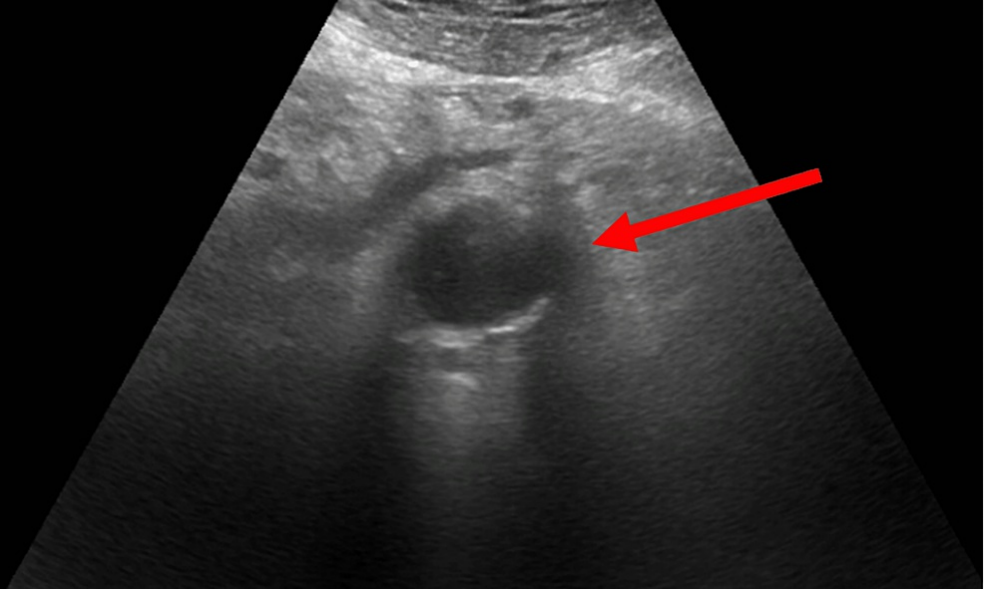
Sonogram depicting an asymmetric outpouching of the aortic wall without an intimal flap
